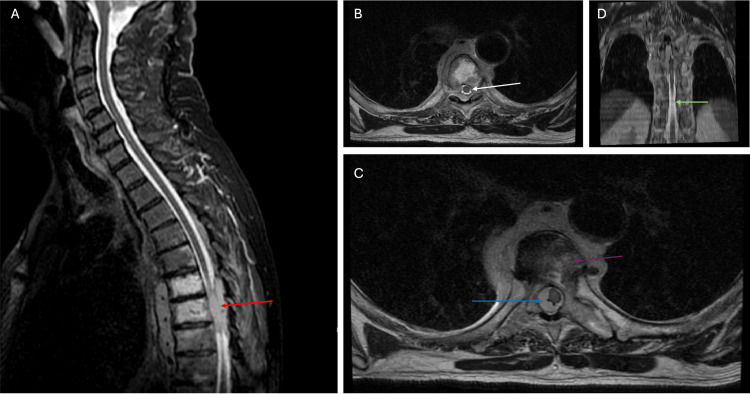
T2-weighted MRI images of the thoracic spine demonstrating pathological infiltration of the vertebrae and associated MSCC at the level of T6, T7, and T8 A: Sagittal T2-weighted MRI image showing an infiltrative lesion of the mid-thoracic vertebral bodies with extension into the posterior epidural space (red arrow). The mass results in marked effacement of the cerebrospinal fluid (CSF) space and flattening of the spinal cord. The heterogeneous T2 signal of the involved vertebral bodies is consistent with vertebral body infiltration from malignant disease. B: Axial T2-weighted MRI image at the level of compression demonstrating loss of a uniform bright CSF rim around the spinal cord (white arrow), in addition to a flattened and displaced spinal cord. C: Axial T2-weighted slice slightly inferior to panel B, showing soft tissue infiltration (blue arrow), continued deformation of the spinal cord, and loss of CSF space. Continued abnormal marrow signal within the vertebral body (purple arrow) is consistent with osseous involvement by malignant disease. D: Coronal T2-weighted MRI image allowing localisation of the lesion (green arrow). MSCC: Metastatic spinal cord compression
